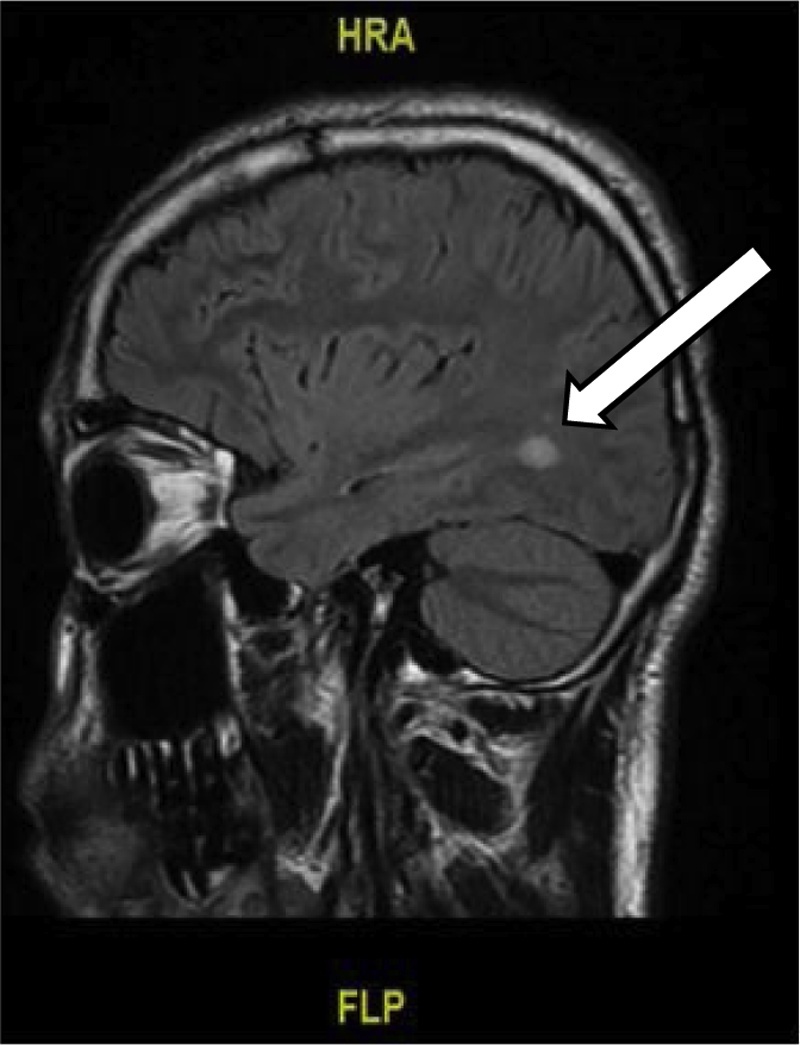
Sagittal view MRI brain showing T2/FLAIR hyperdensity in the left temporal-occipital white matter. This non-specific finding can be seen with demyelinating disease or rarely trauma.
